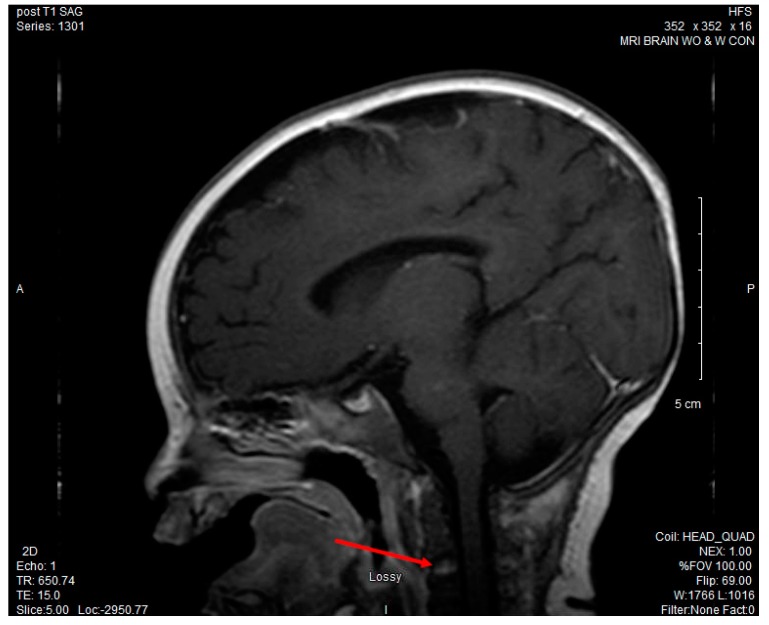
Magnetic resonance imaging (MRI) brain with and without contrast: increased T2 enhancement of the C3 vertebral body concerning for osteomyelitis.
